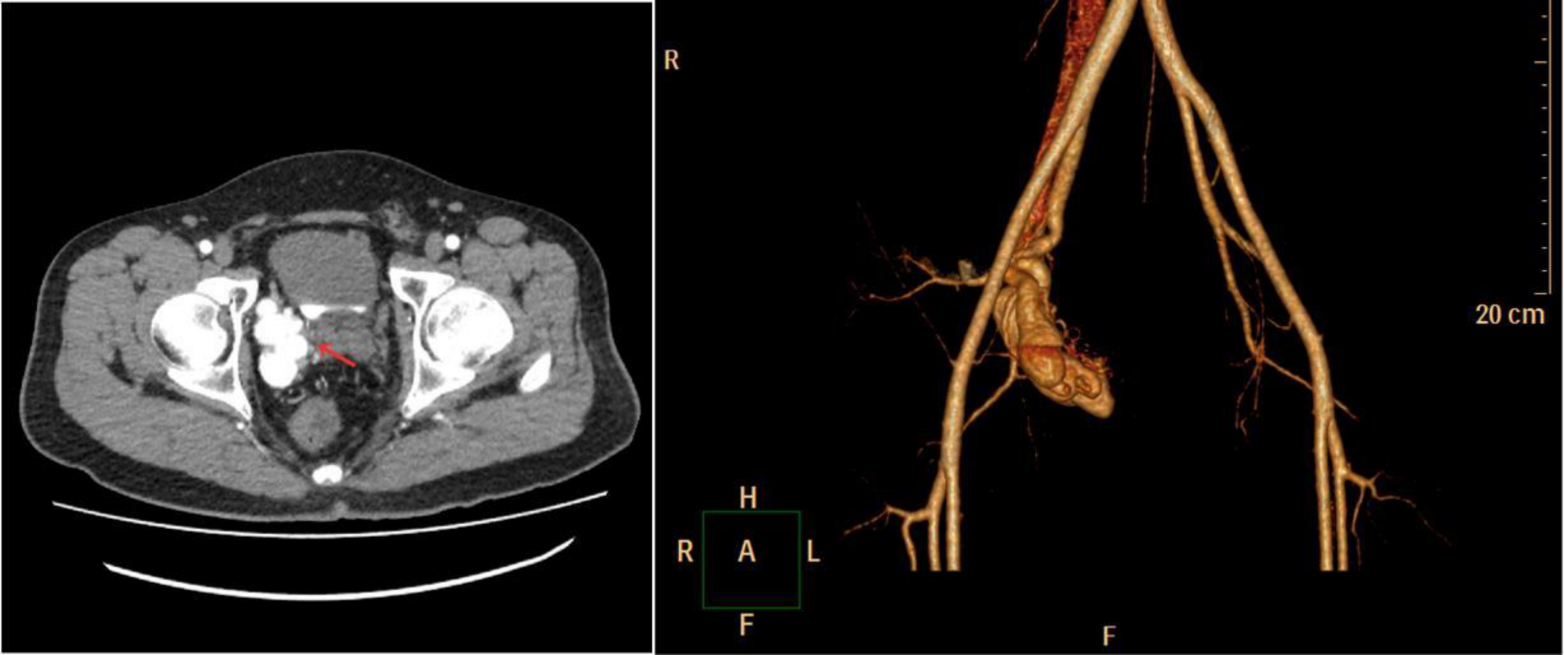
Computed tomographic angiography showed a large pelvic arteriovenous malformation (red arrow) supplied mainly by the right internal iliac artery.
